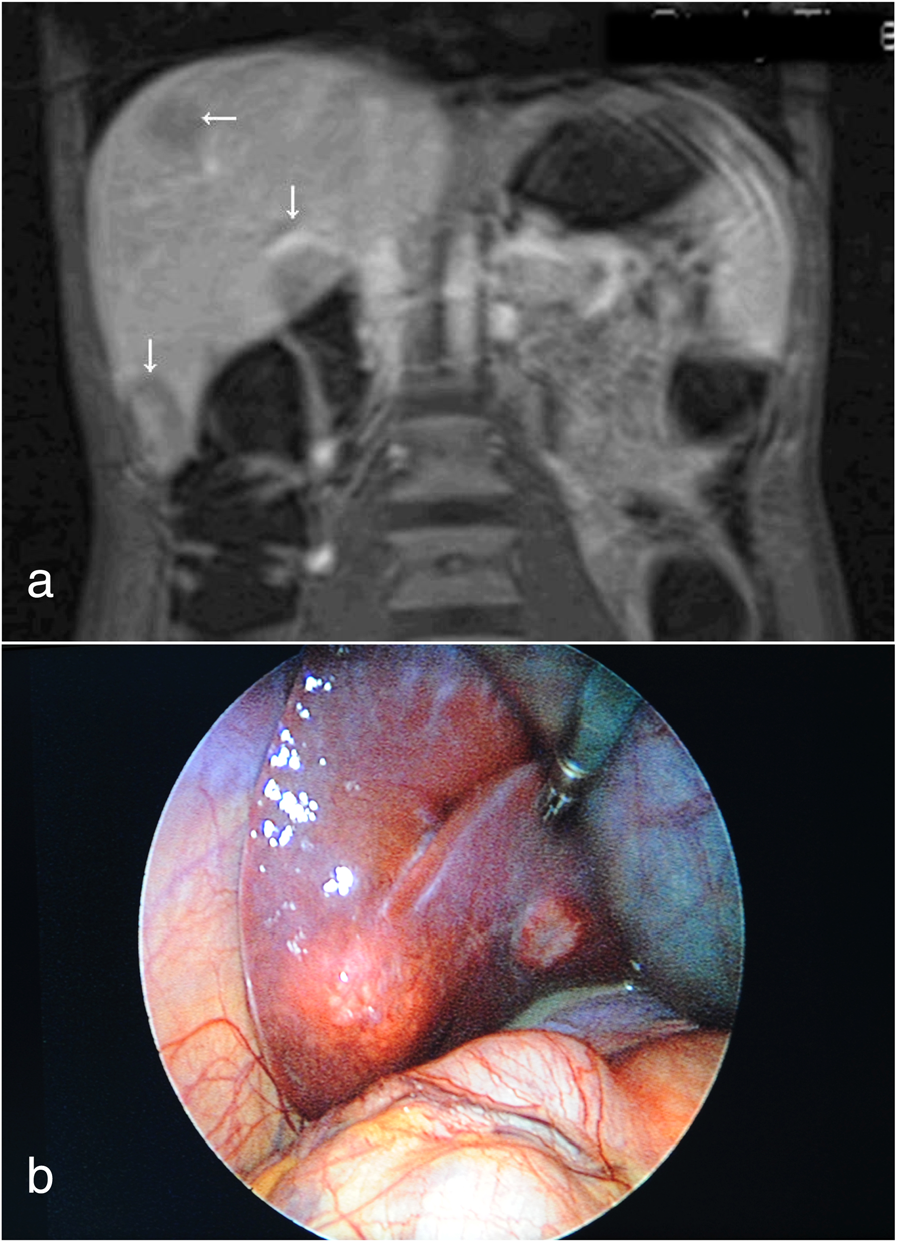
Liver metastases. a T1-weighted hydromagnetic resonance imaging scan of the patient‘s abdomen (Gadovist coronary contrast agent; Bayer HealthCare, Berlin, Germany) shows multiple hypodense liver lesions (arrows). b Photograph taken during laparoscopy, showing one of the liver metastases
